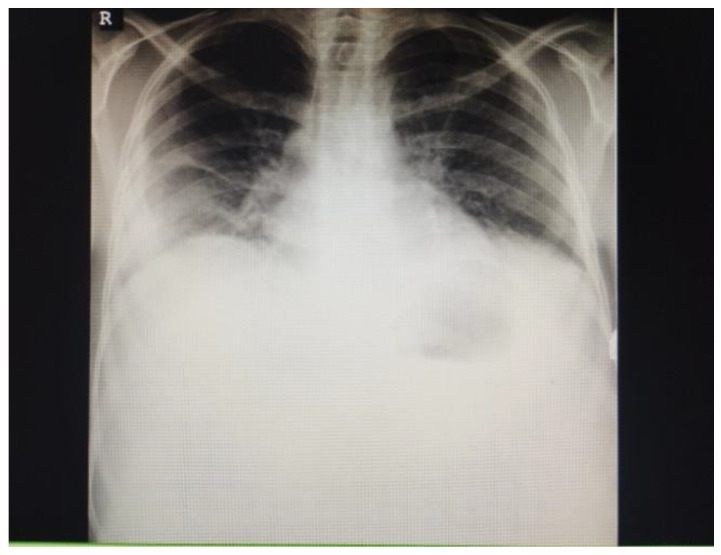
Chest radiograph. Right axillary and basal inflammatory infiltrates. R-right side.
